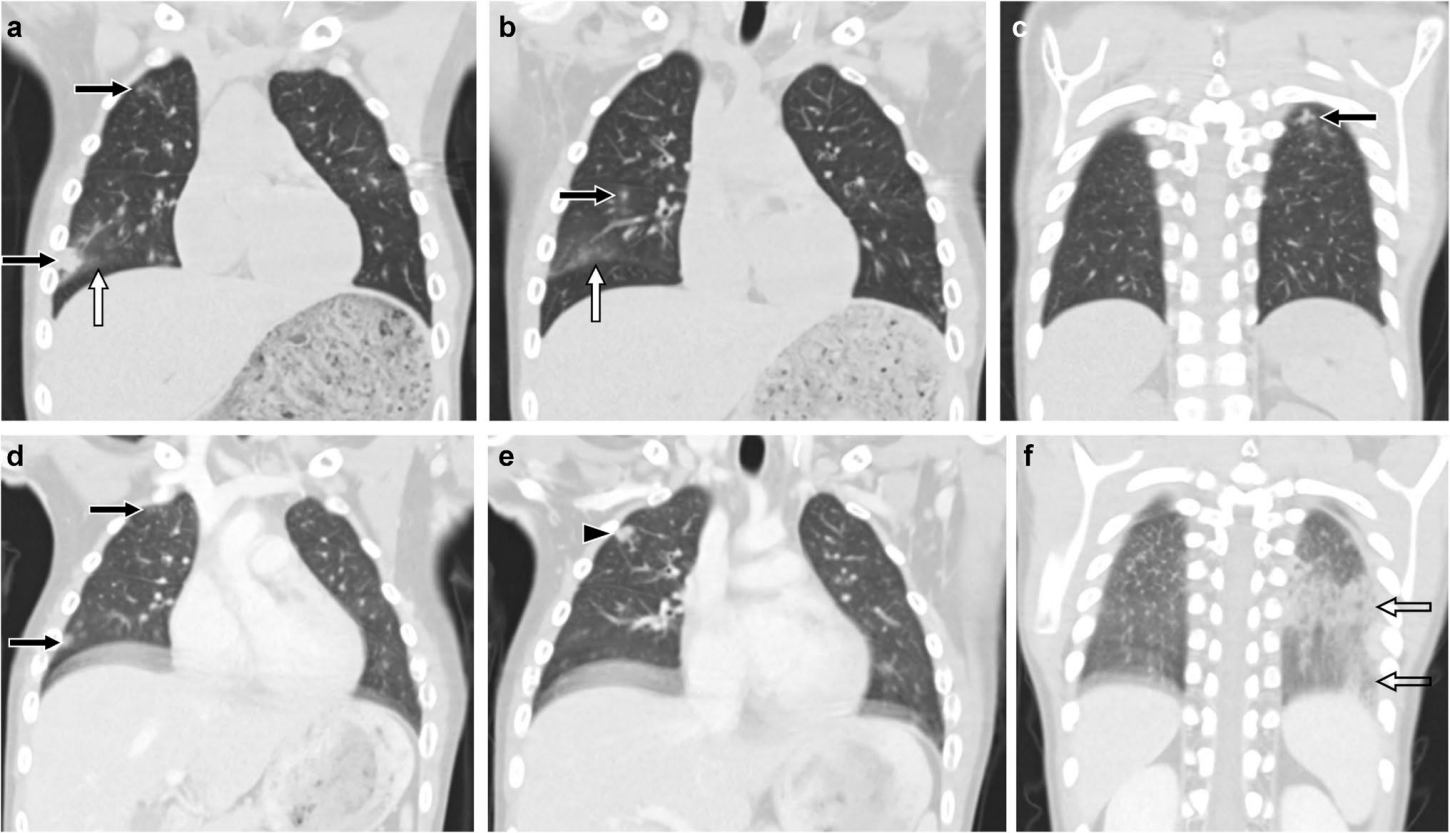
A 10-year-old male receiving sirolimus for tuberous sclerosis complex who presented with fever, achiness, elevated inflammatory markers, and multifocal opacities on chest radiograph in the setting of an elevated sirolimus level of >60 ng/dl (above maximum limit of detection; target ~30 ng/dl for this patient). a-c Coronal CT images without contrast obtained at the time of admission showing multiple bilateral ill-defined nodules (*black arrows*) and ground-glass opacity (*white arrow*). d-f Coronal CT images with contrast obtained 8 days later showing decreased size of previously seen nodules (*black arrows*) and development of new nodules in the right upper lobe (*arrowhead*) as well as consolidation and ground-glass opacity in the left lower lobe (*open arrow*) (i.e., waxing and waning lung findings). Some of the nodules also remained unchanged in size (not shown). Extensive work-up for infectious, oncologic, and rheumatologic etiologies was unrevealing, fever resolved with withholding of sirolimus and initiation of steroids, and chest CT findings had resolved on follow-up imaging obtained approximately 7 weeks later supporting a diagnosis of sirolimus-induced lung disease
